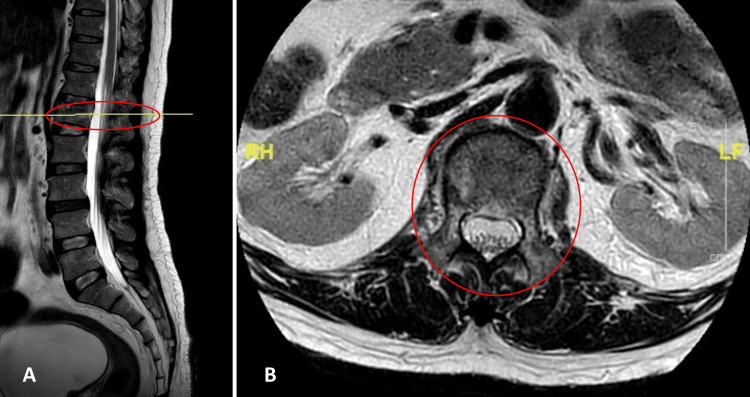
A: Sagittal view indicates the spinal cord level where the MRI was taken. B: T2-weighted MRI of the thoracolumbar spine shows no significant cord edema or changes in fracture morphology MRI: magnetic resonance imaging
